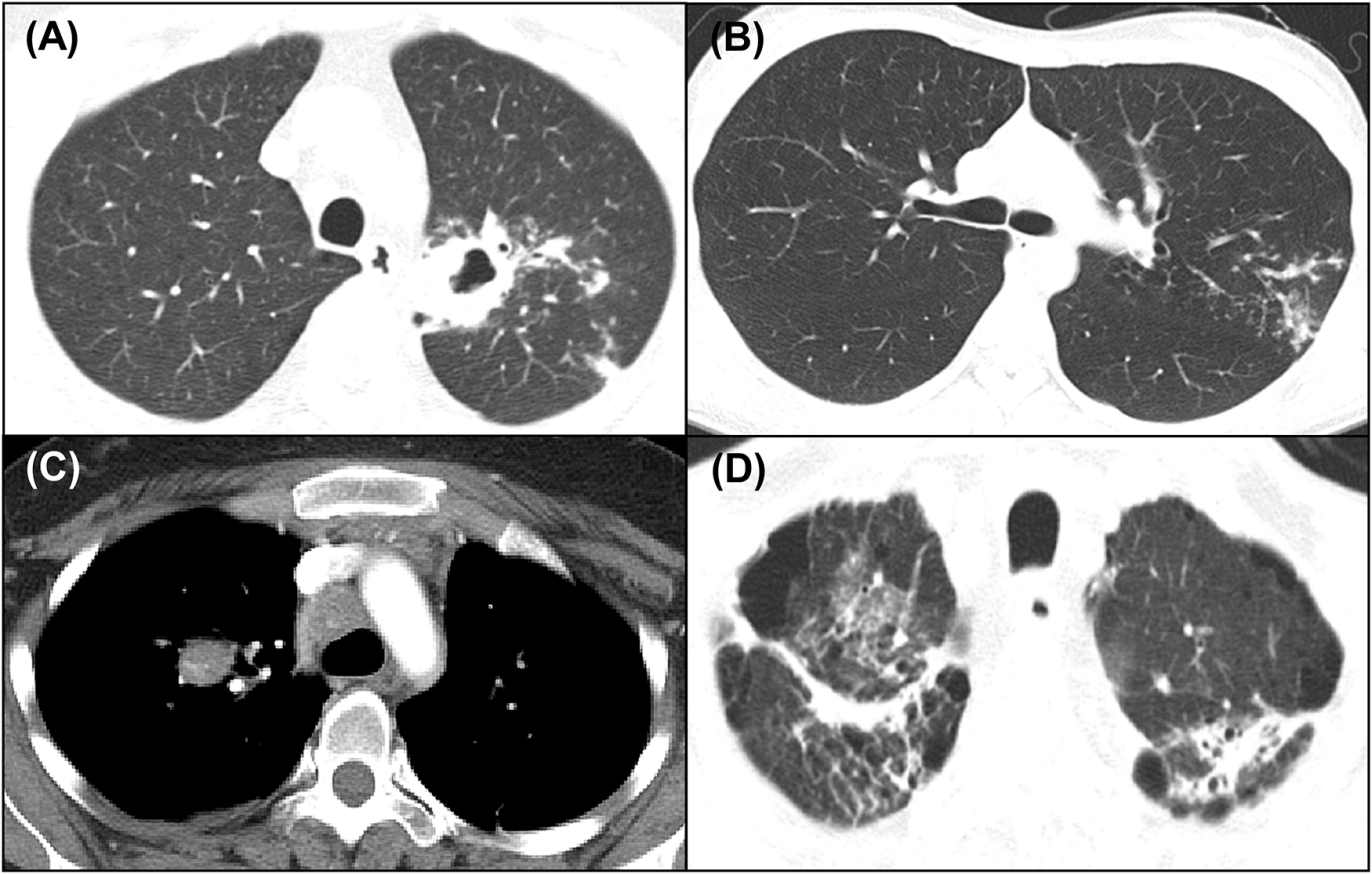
The representative cases according to chest CT classification. (A) definitely-active, with lesions including a cavity (B) probably-active, showing a “tree-in-bud” appearance or multiple non-calcified poorly circumscribed nodules without a cavity (C) indeterminate-activity, with lesions appearing mainly as non-calcified well-circumscribed nodules (D) probably-inactive, with lesions appearing mainly as calcified nodules or fibrotic bands.
